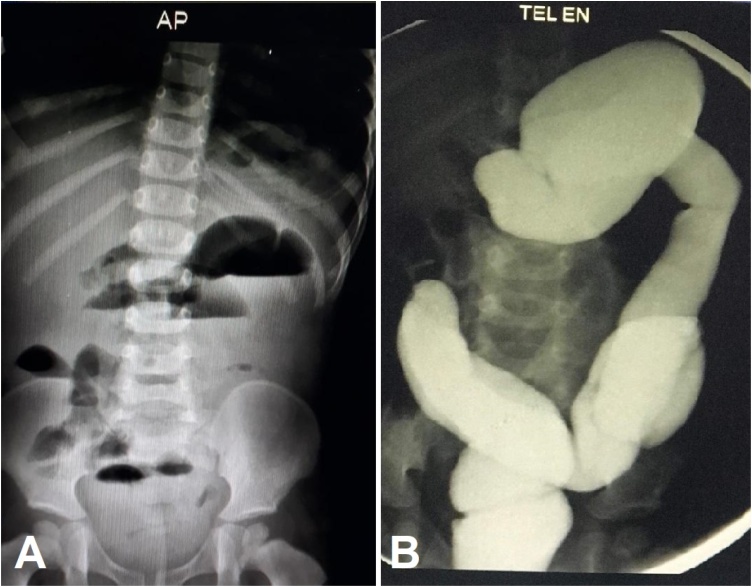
Initial radiological imaging for the child (A) Plain abdominal x-ray shows multiple air-fluid levels, and (B) Barium enema shows a complete obstruction in the mid transverse colon.
